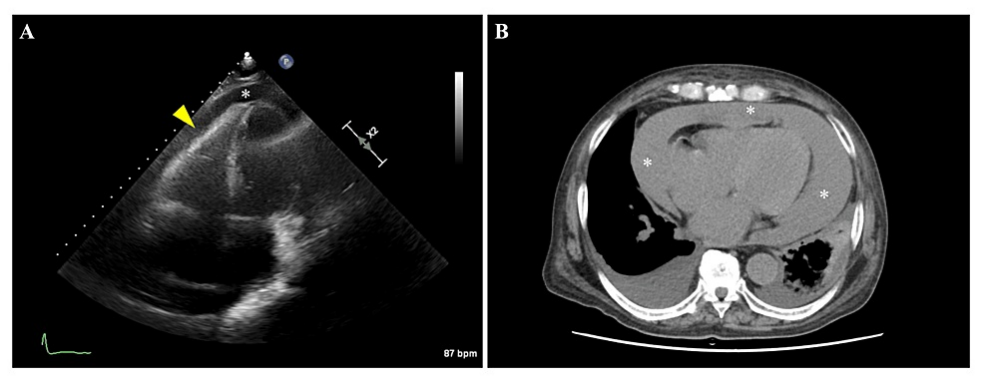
Transthoracic echocardiography and computed tomography (A) A four-chamber image of transthoracic echocardiography revealed a large pericardial effusion (asterisk) and compression of the right ventricle in early diastole (yellow triangle). (B) A computed tomography (CT) scan revealed significant pericardial effusion (asterisks). The CT value of pericardial effusion was 35 Hounsfield units, suspected to be hematogenous, whereas that of pleural effusion was 10 Hounsfield units.
